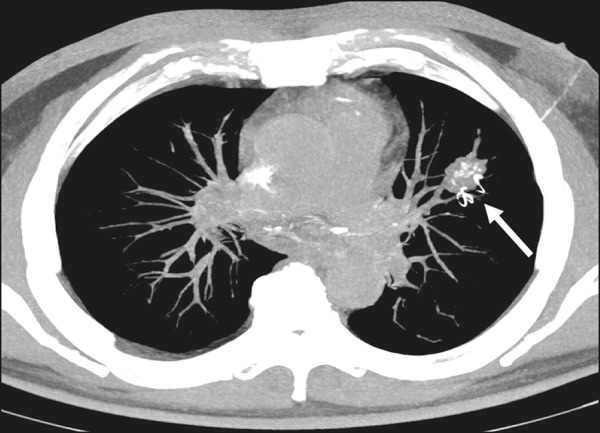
Axial MIP CT reconstruction showing the hookwire positioning, adjacent to
